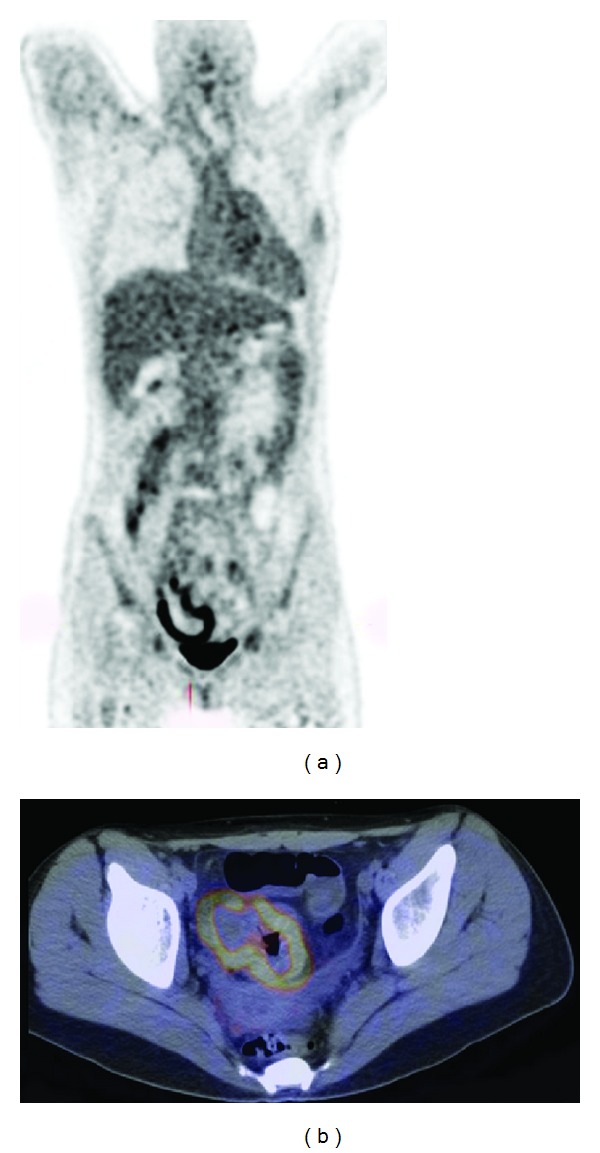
A 14-year-old girl known with Crohn's disease. FDG-PET/CT (left: MIP image of the FDG-PET, right: fused FDG-PET/CT transaxial slice) showed inflammation of the caecum.
